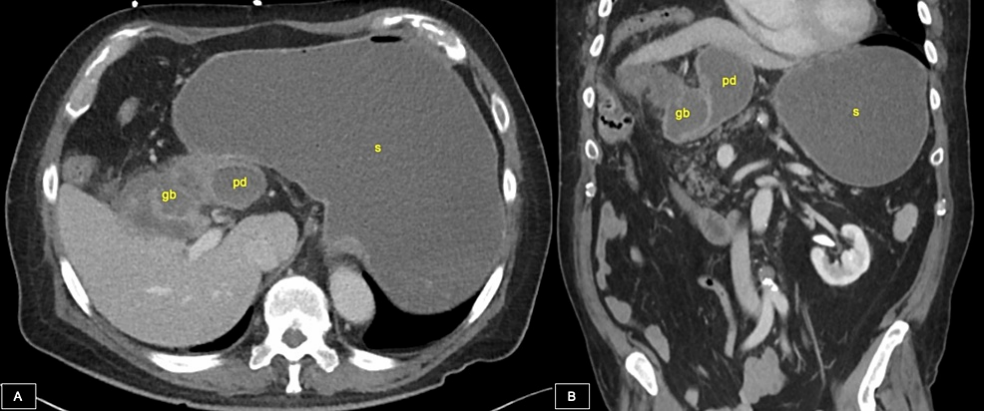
Computed tomography (CT) imaging findings 1a: Axial CT imaging of the abdomen with intravenous (IV) contrast showing massively distended stomach (s) and proximal duodenum (pd) with irregular gallbladder (gb) closely approximated to the proximal duodenum. 1b: Coronal CT imaging of the abdomen with IV contrast demonstrating compression of the proximal duodenum (pd) by the gallbladder.
